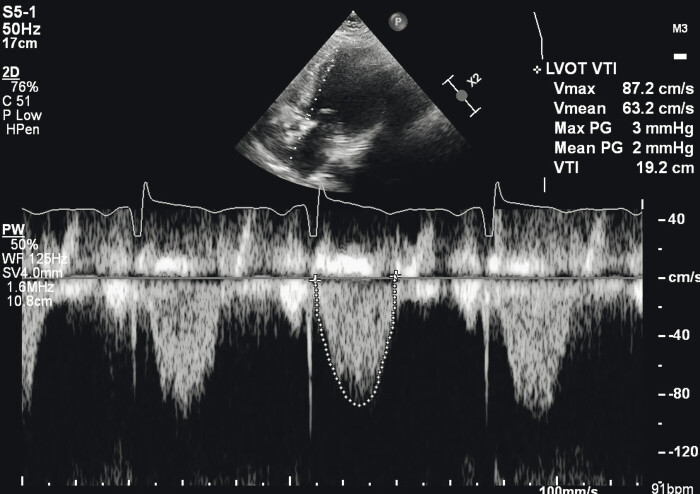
Transthoracic echocardiography demonstrating severe calcification of a bicuspid aortic valve with critical aortic stenosis, moderate left ventricular systolic dysfunction, regional wall motion abnormalities, and concentric left ventricular hypertrophy.
